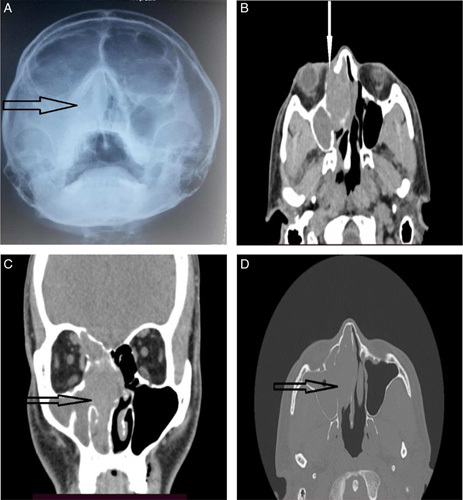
Plain Radiograph of PNS- Water’s view (A), Axial section image of CT PNS soft tissue window (B), Coronal section image of CT PNS soft tissue window (C), Axial section image of CT PNS Bone window (D). PNS, paranasal sinuses.
